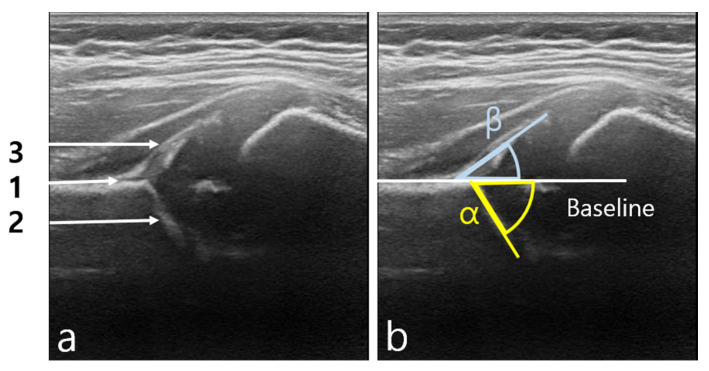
(a) A standard hip ultrasonographic (US) image should show three key anatomical structures: 1. vertical ilium, 2. lower margin of Os ilium, and 3. labrum. (b) Alpha (α) and beta (β) Graf angles in a US image of the hip.
